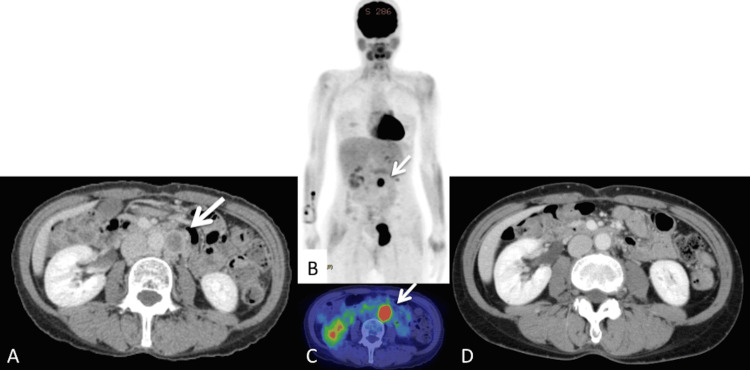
CT scan and PET/CT of a re-enlarged left para-aortic node after surgery and chemotherapy (progressive disease) (A, B, C; arrow). SUVmax of the node was 9.14, and its short axis was 25 mm. After radiotherapy, the enlarged node decreases in size and is considered normal (complete response) (D).
